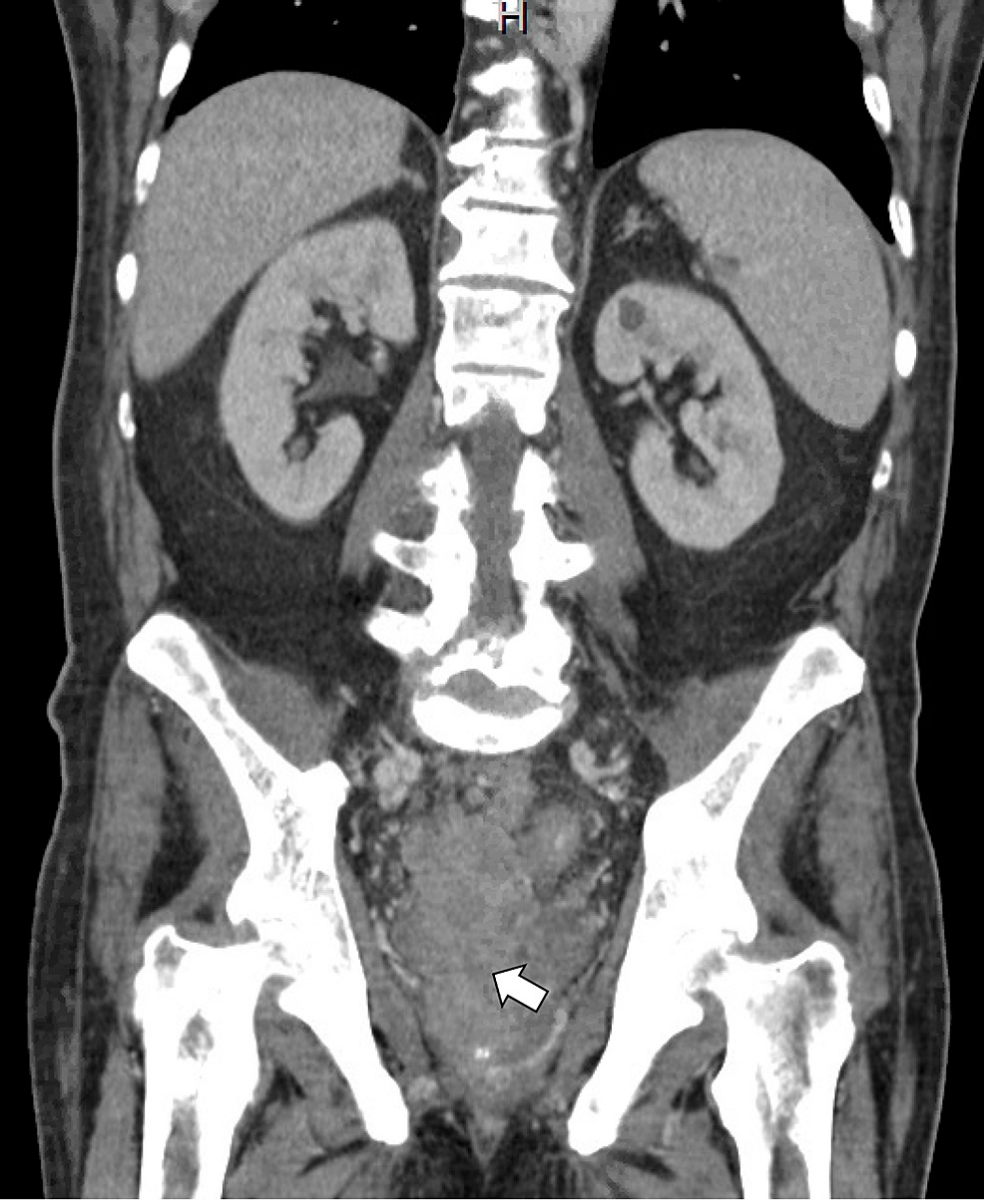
CT scan (coronal plane) of the abdomen and pelvis White arrowhead shows tumor arising from the prostate invading the pelvis CT, computed tomography
